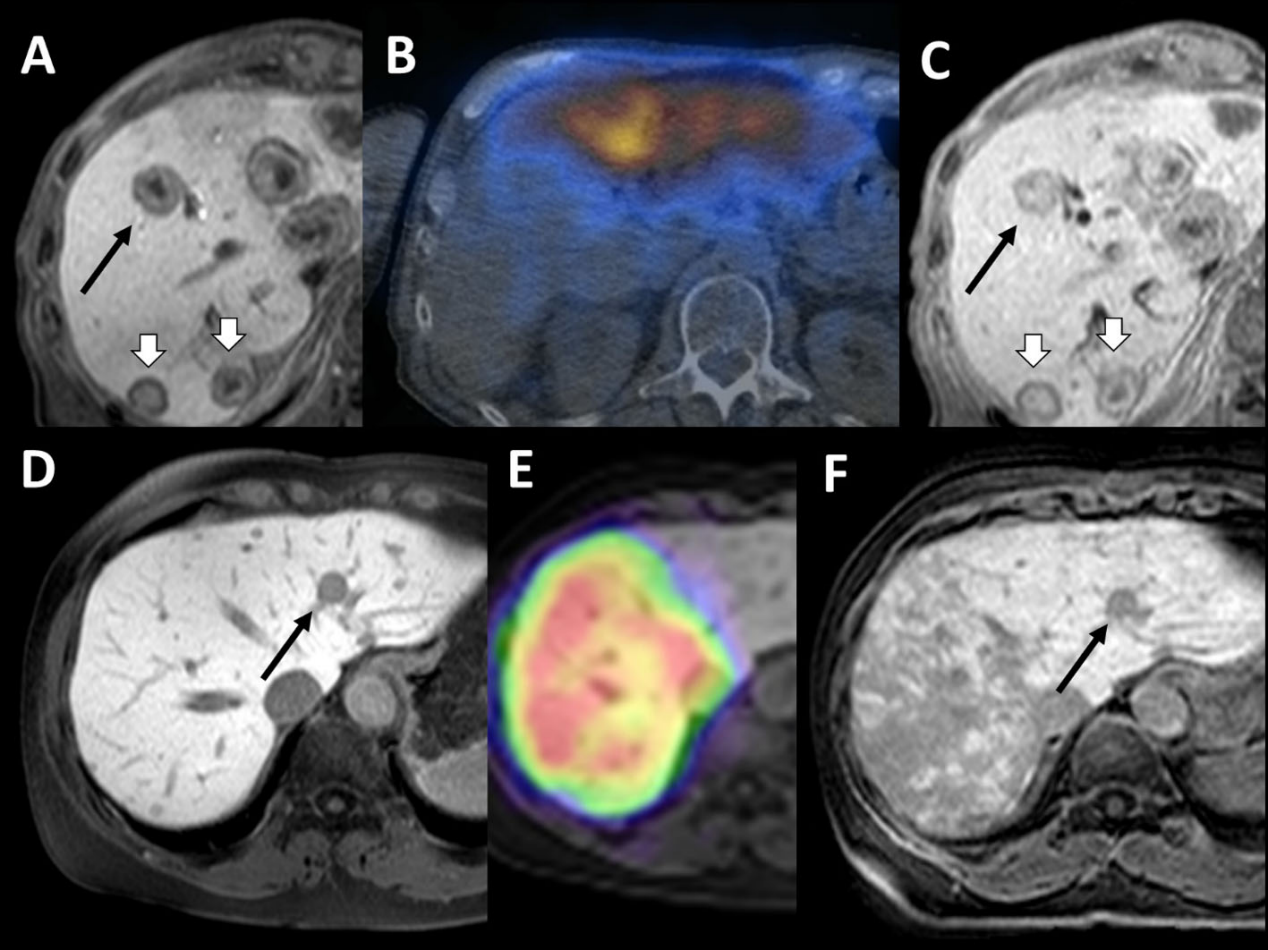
Two cases of size regression < 30%, but noticeably smaller, A–C nasopharyngeal carcinoma, D–F breast cancer: A and C) black arrow show a metastasis decreasing from 22 to 20 mm (i.e., 10%), white arrows indicate progressing lesions, e.g., 18–20 mm and 19–22 mm. B Bremsstrahlung-SPECT/CT examination: RE left and segment 4b. Untreated segments 5–8 and 4a. D and E black arrow: 12–10 mm (~ 20%). F Radiation damage in the liver, typical RE image for breast cancer
